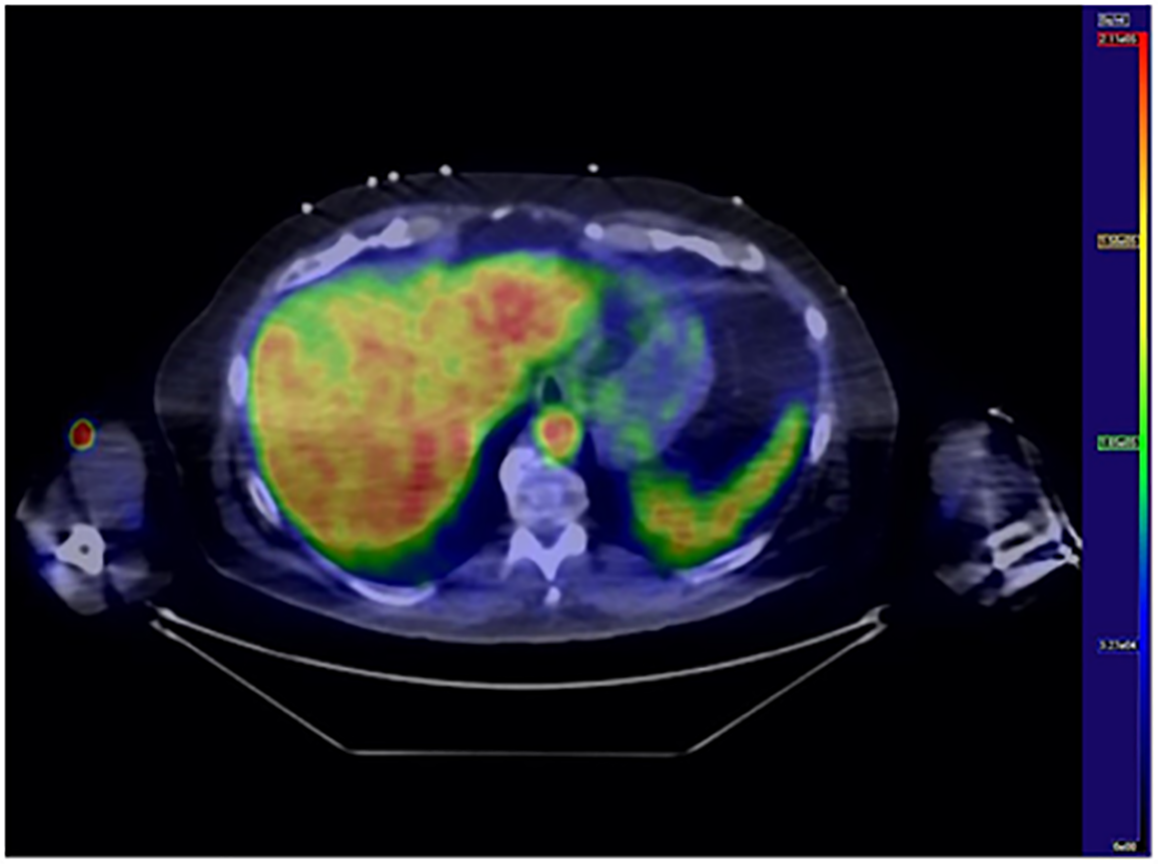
Hepatic glucose uptake. Transaxial [^18^F]FDG PET/CT image of the liver. Blue color represents low [^18^F]FDG uptake whereas red color represents high uptake.
